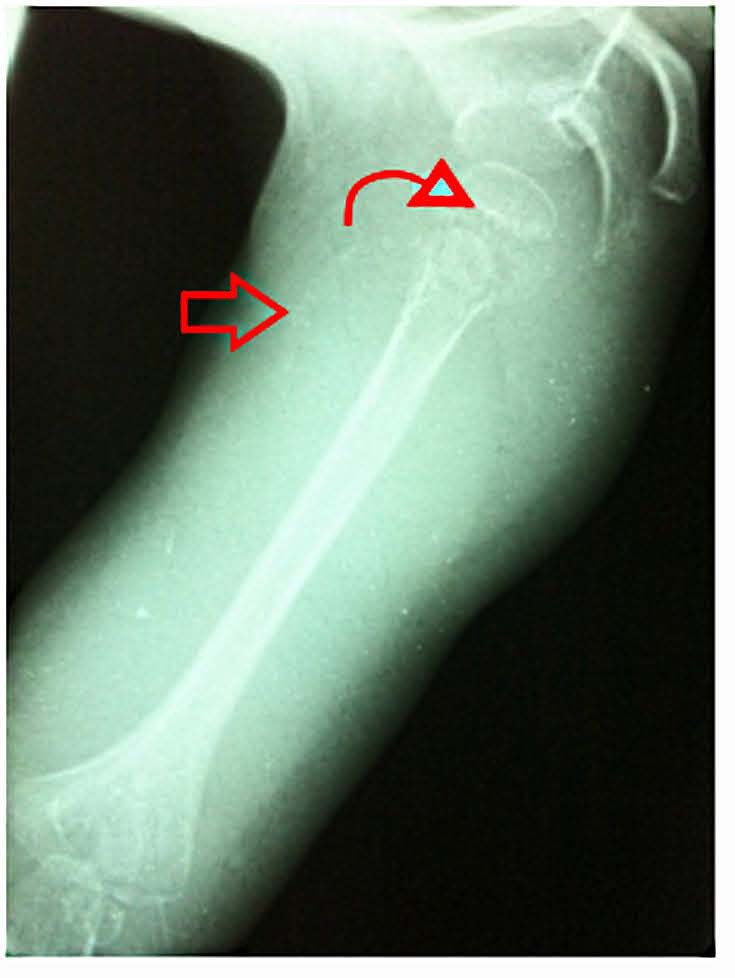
Radiograph of right shoulder shows dense, linear calcification in the distal metaphysis “white line of Frankel” (curved arrow) and periosteal separation (red arrow).
